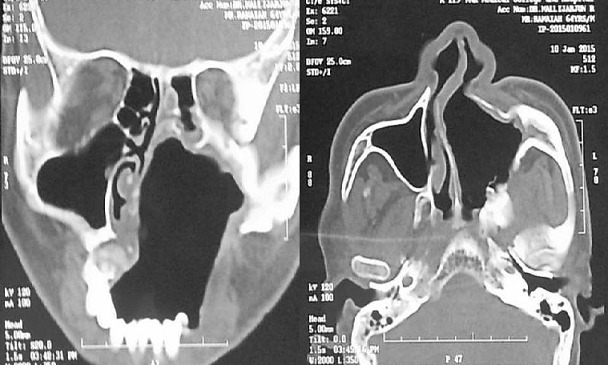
Computed tomography image sagittal and coronal section showing irregular radiolucency suggestive of post operative left maxillectomy, deviated nasal spine to right, left ethmoidal and sphenoidal sinusitis, Changes seen involving left pterygoid plates and posterior wall of maxillary antrum suggestive of residual changes of Osteomyelitis
